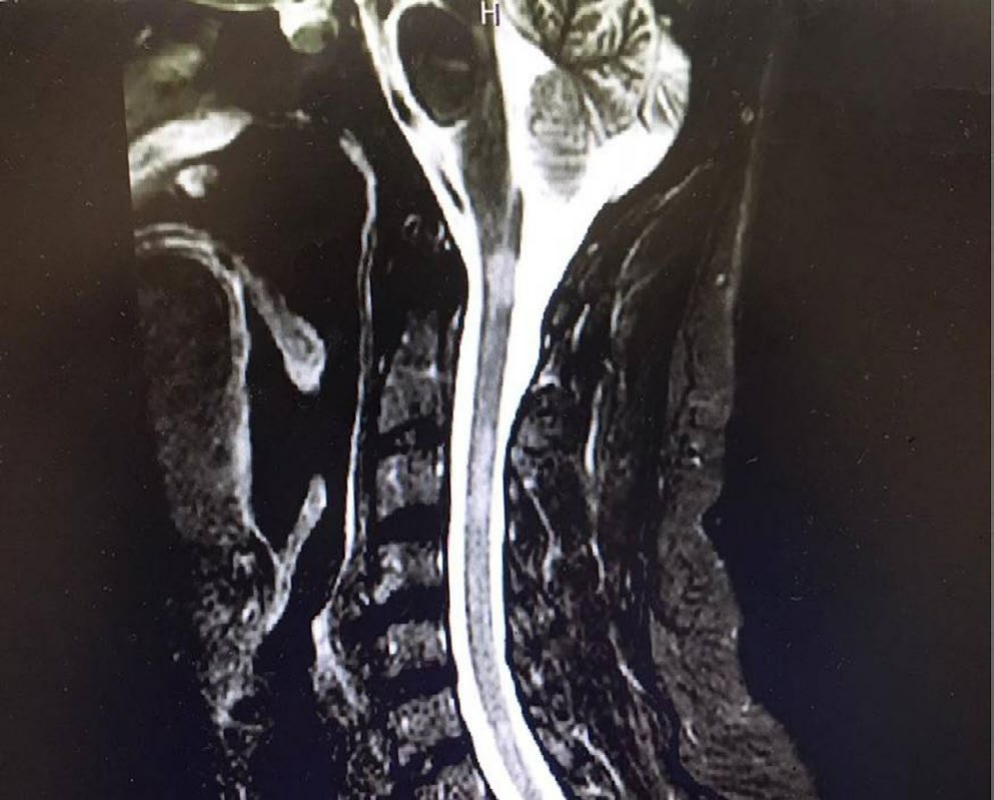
T2‐STIR cervical MRI shows multiple bright lesions compatible with demyelinating disease (multiple sclerosis)
